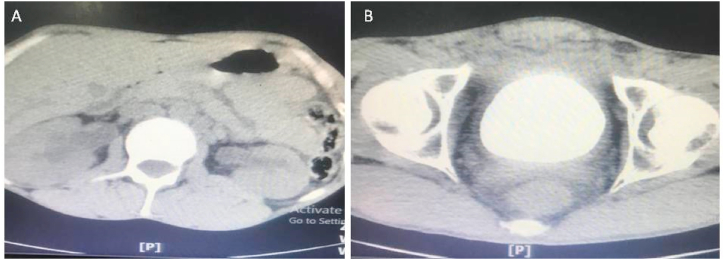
CT scan without contrast showed: A-mild to moderate bilateral hydronephrosis, B- a large urinary calculus in the bladder.
